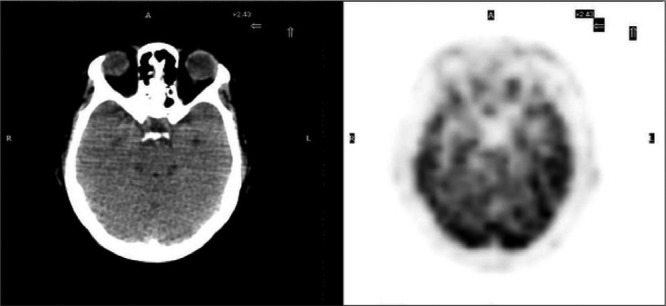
Axial CT and PET images of the orbita in the first PET/CT scan
